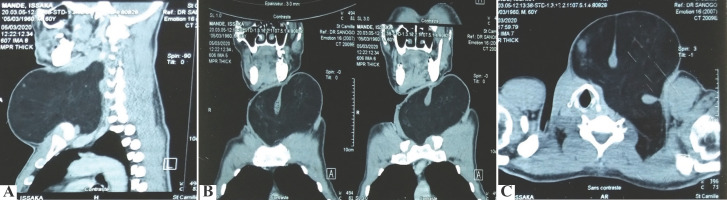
Tomodensitométrie cervico-thoracique : masse hypodense baso-antéro-latérale gauche bien limitée évoquant un lipome géant 2a : coupe sagittale 2b : coupe coronale 2c : coupe axiale Cervicothoracic CT scan: well-limited left anterolateral basal hypodense mass evoking a giant lipoma 2a: sagittal section 2b: coronal section 2c: axial section
